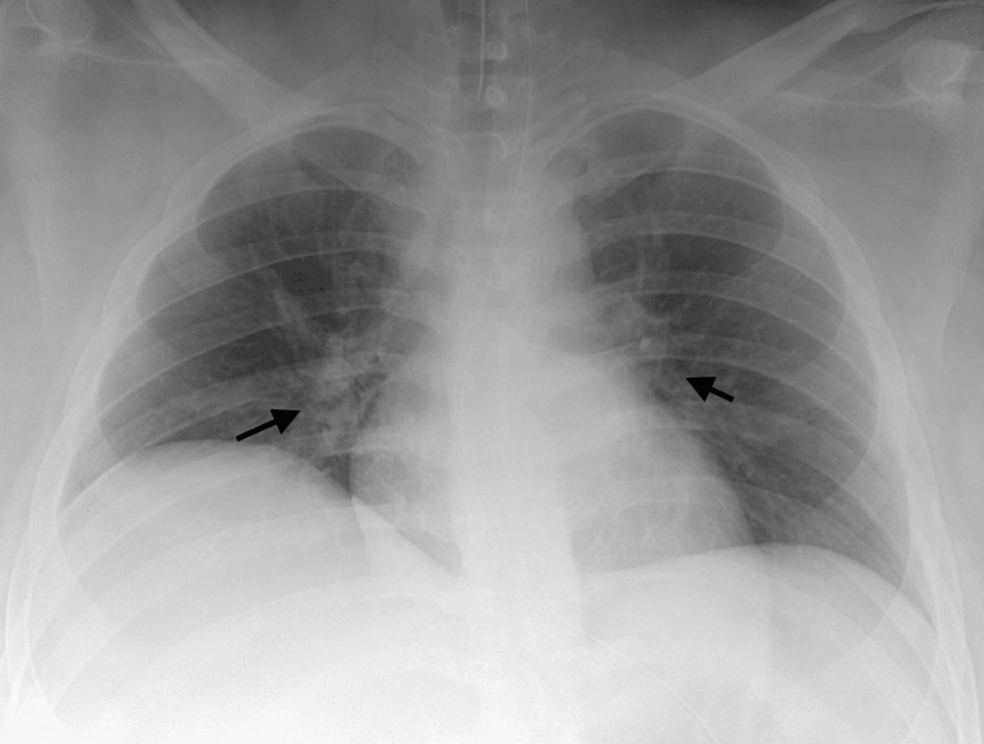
Case 3 chest X-ray Arrows indicating bilateral pulmonary infiltrates
